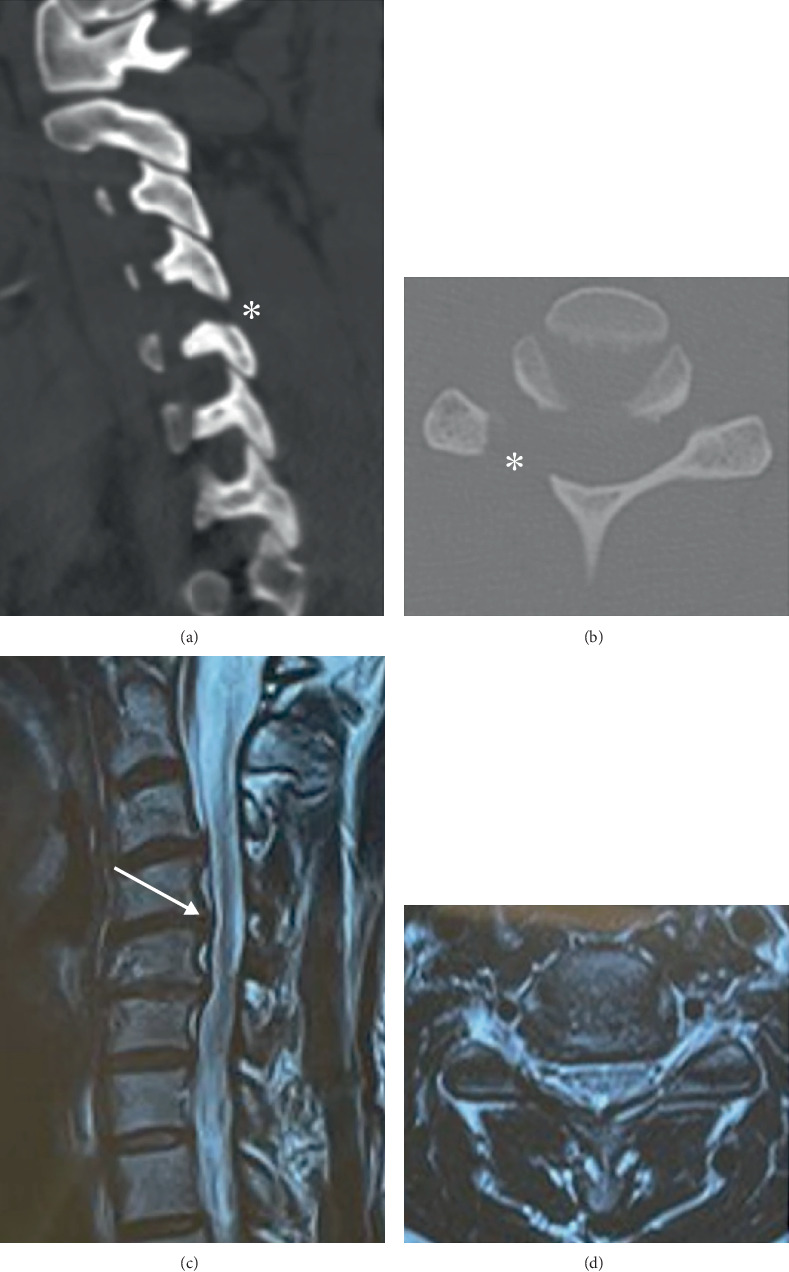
Postoperative exams. (a, b) CT showing satisfactory right C4–C5 foraminotomy (asterisk). (c, d) MRI with reduction of the herniated volume (arrow).
